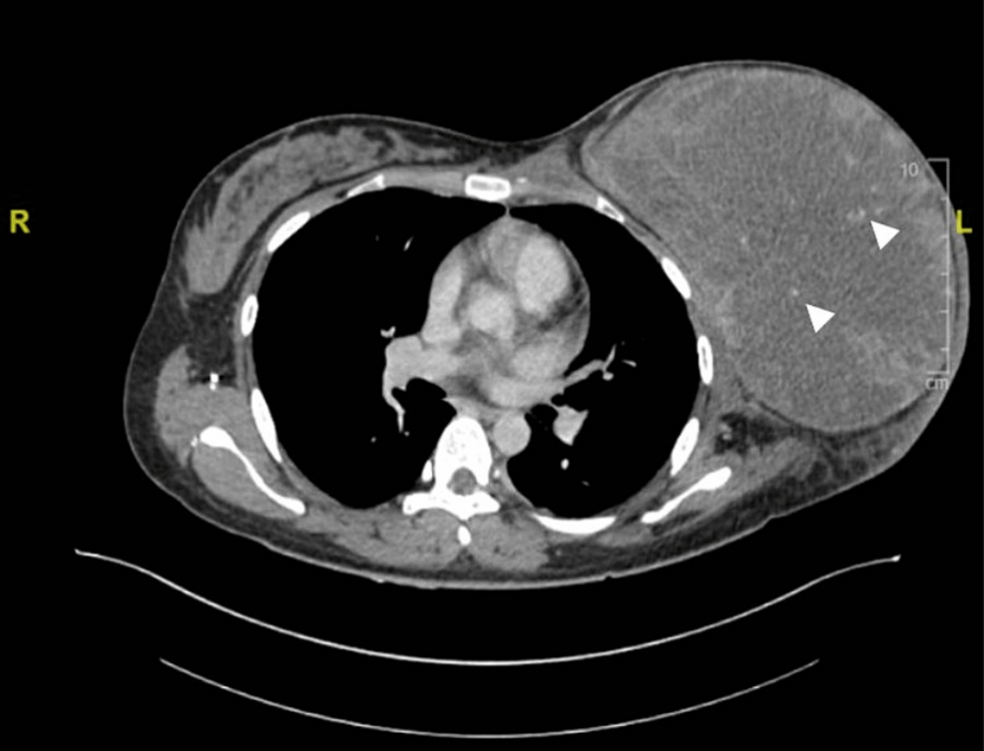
Contrast-enhanced computed tomography of the thorax showed a large, well-defined, heterogeneously enhancing left breast mass with central non-enhancement suggestive of necrosis and foci of calcification within (arrowheads).
